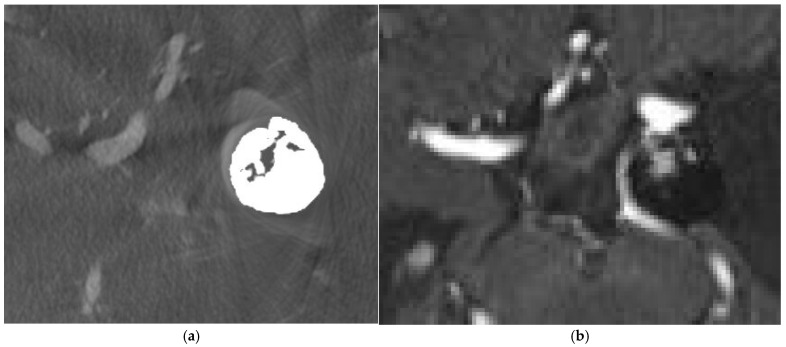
Coil embolization for left posterior communicating artery (PCoA) aneurysm. (a) IV CBCT with metallic artifact reduction (MAR) showed coil mass in left PCoA aneurysm with areas of loose packing or coil impaction, but no definite contrast enhancement was observed within aneurysmal sac. (b) Contrast-enhanced MRA showed contrast enhancement within coiled left PCoA aneurysmal sac, suggestive of slow residual flow.
